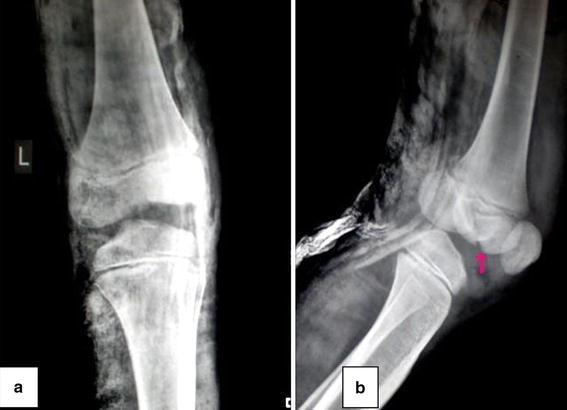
Preoperative anteroposterior a and lateral b radiographs of the case. The *arrow* in the lateral view indicates the fracture line
